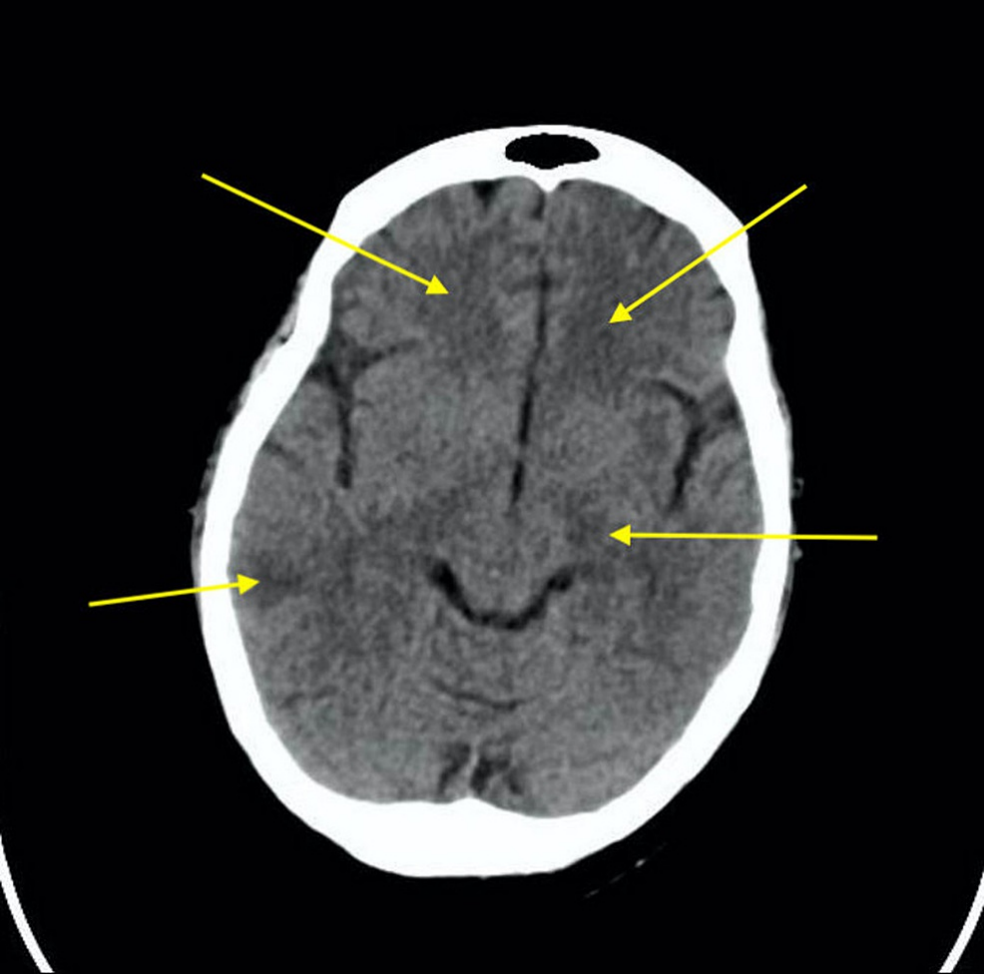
Non-contrast CT of the brain showing axial cuts at the basal ganglia Yellow arrows display the hypodensities in the right parietal lobe, supratentorial, and infratentorial regions.
